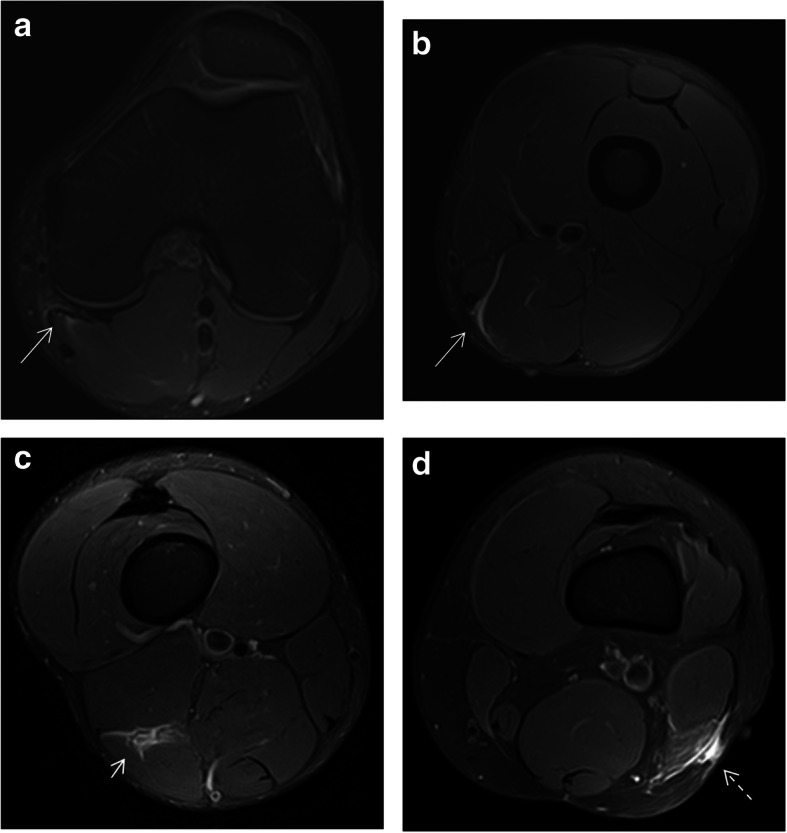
Axial MR images in fluid-sensitive sequences in distal myoconnective injuries of the semimembranosus muscle showing muscular edema (arrows) at different levels (a and b). Edema in the deep (short arrow, c) and superficial (dashed arrow, d) zippers of the femoral biceps
